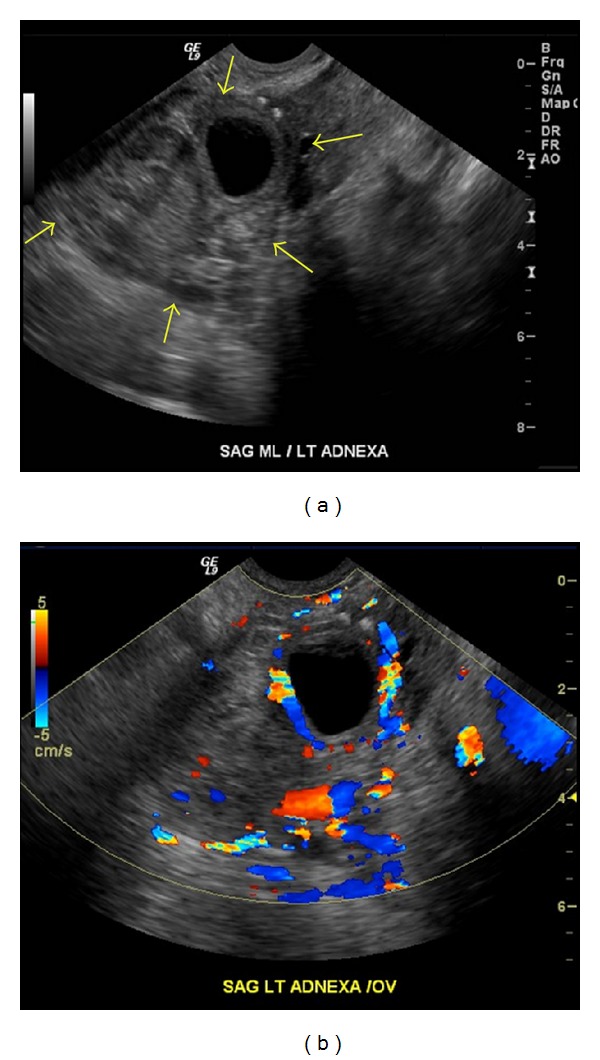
Image (a) is a transvaginal sagittal US image through the left adnexal region which demonstrates a heterogeneous mass (arrows) containing an anechoic cystic structure with slightly echogenic thick walls. Image (b) is a Doppler US image through the same area demonstrating an intense peripheral vascularity with a “ring of fire” appearance.
